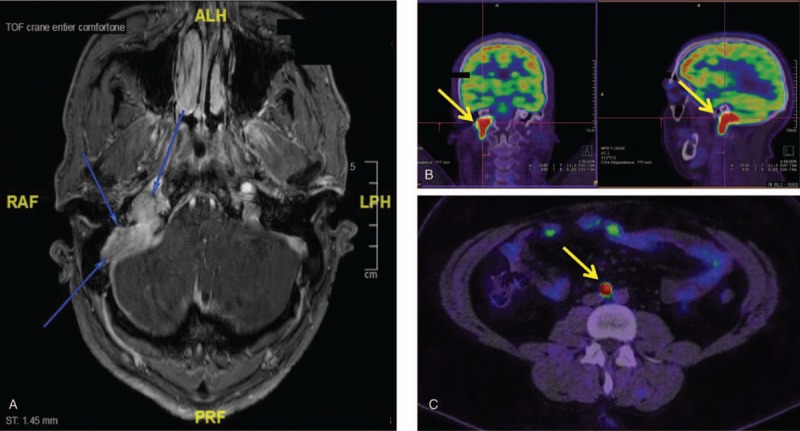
A: MRI showed a large PGL at the right posterior jugular foramen. B: High uptake on FDG-PET revealing a large PGL at the right posterior jugular foramen. C: FDG-PET: High uptake of an inter-aortico-cave lesion, compatible with a PGL. MRI = magnetic resonance imaging, PGL = paraganglioma.
